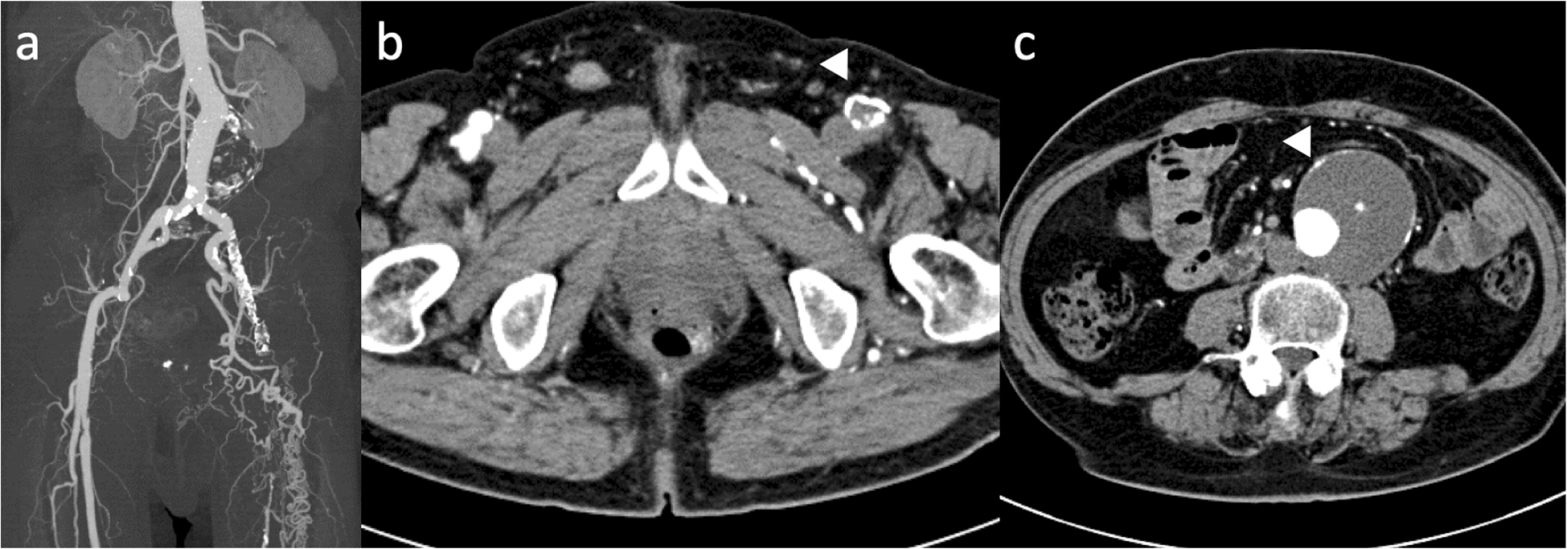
CT before endovascular aneurysm repair. a: MIP image of CT angiography shows the occlusion of left EIA to SFA with abundant collateral channels from left hypogastric artery, b: transaxial image shows the occluded CFA with circumferential vessel wall calcification (arrow head), c: abdominal aortic aneurysm with the diameter of 55 mm (arrow head). CFA: common femoral artery, EIA: external iliac artery, MIP: maximum intensity projection, SFA: superficial femoral artery
